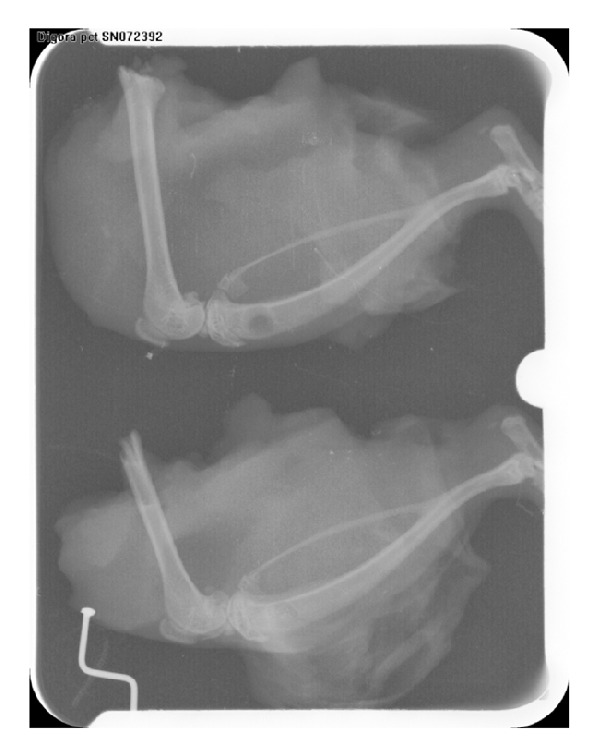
Radiographic view on day 7. A: Left tibia. B: Right tibia.
